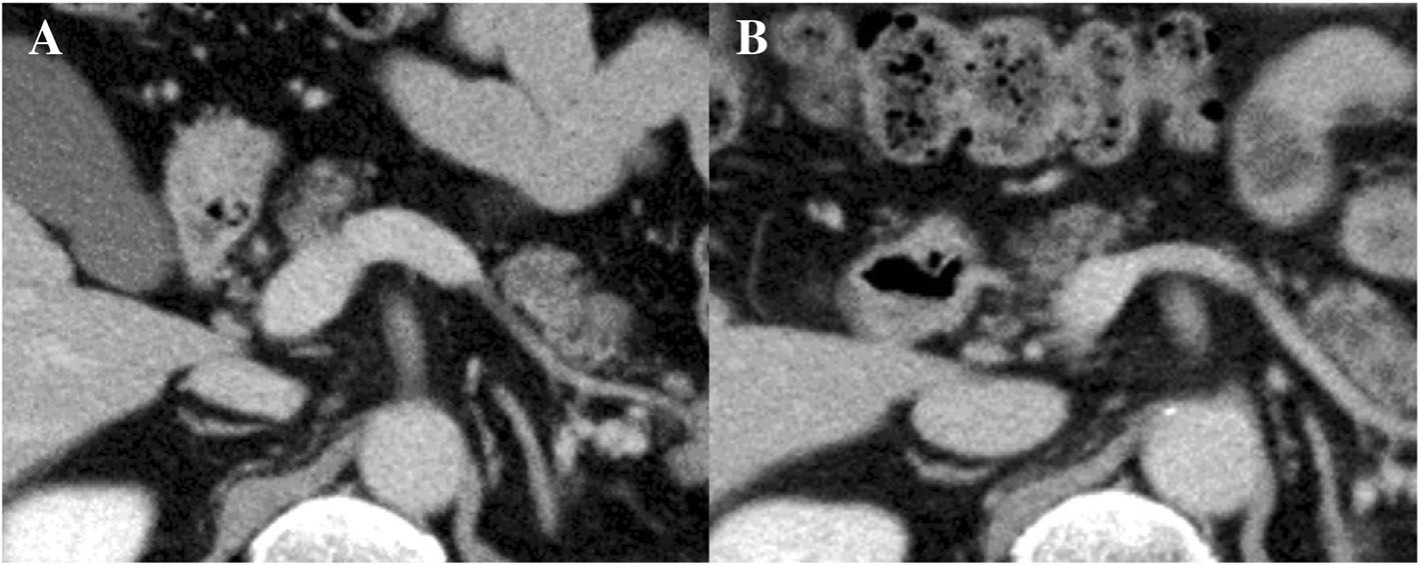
Abdominal CT taken on the postoperative day 28 (b) showed that the diameter of the portal vein was diminished in size compared to its preoperative state (a)
